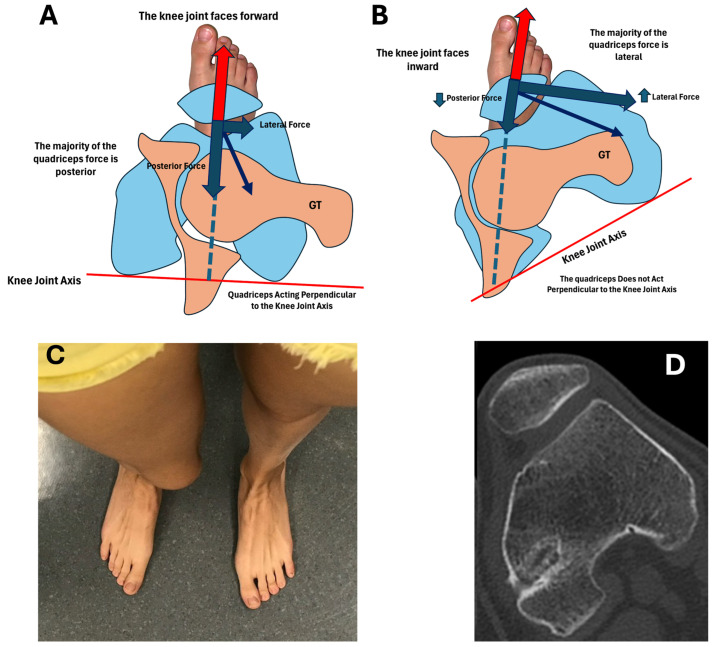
(A) Normal female with femoral anteversion (FAV) of 13° and ETT of 27°. (B) Female with 43° of ETT. To keep the foot progression angle normal, the knee joint axis (red line) points inward causing increased strain on the knee. The hip is markedly internally rotated with the greater trochanter (GT) oriented anteriorly. (C) Female with 43° of right-side ETT. (D) Computed tomography scan of the patient’s right knee shown in image C. The knee joint faces inward. This patient has a FAV of 15° and an ETT of 43°. (C) Republished from reference [15]. Published by Elsevier Inc. on behalf of International Society of Arthroscopy, Knee Surgery and Orthopedic Sports Medicine.
